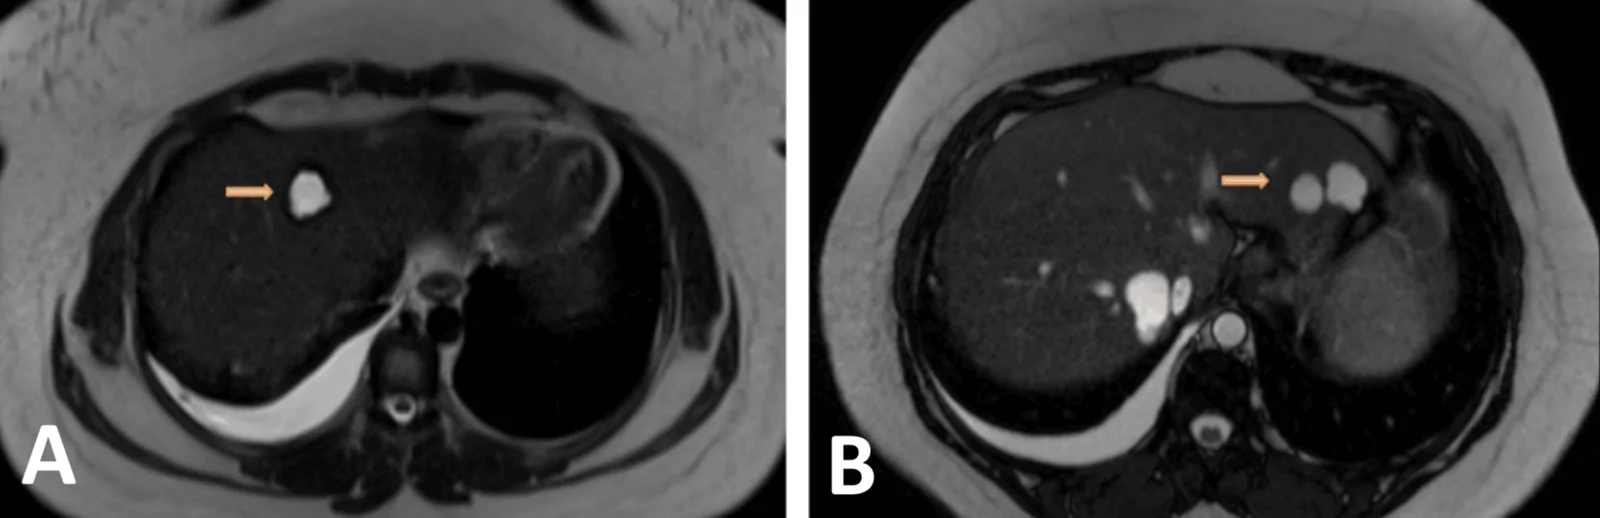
MRI scan of the liver on admission: (A, B) Cross-sectional images of the upper part of the liver showing multiple round, hyperintense nodules in the right and left lobes of the liver, with an associated pleural effusion The arrows indicate round nodules representing cystic changes in the liver.
